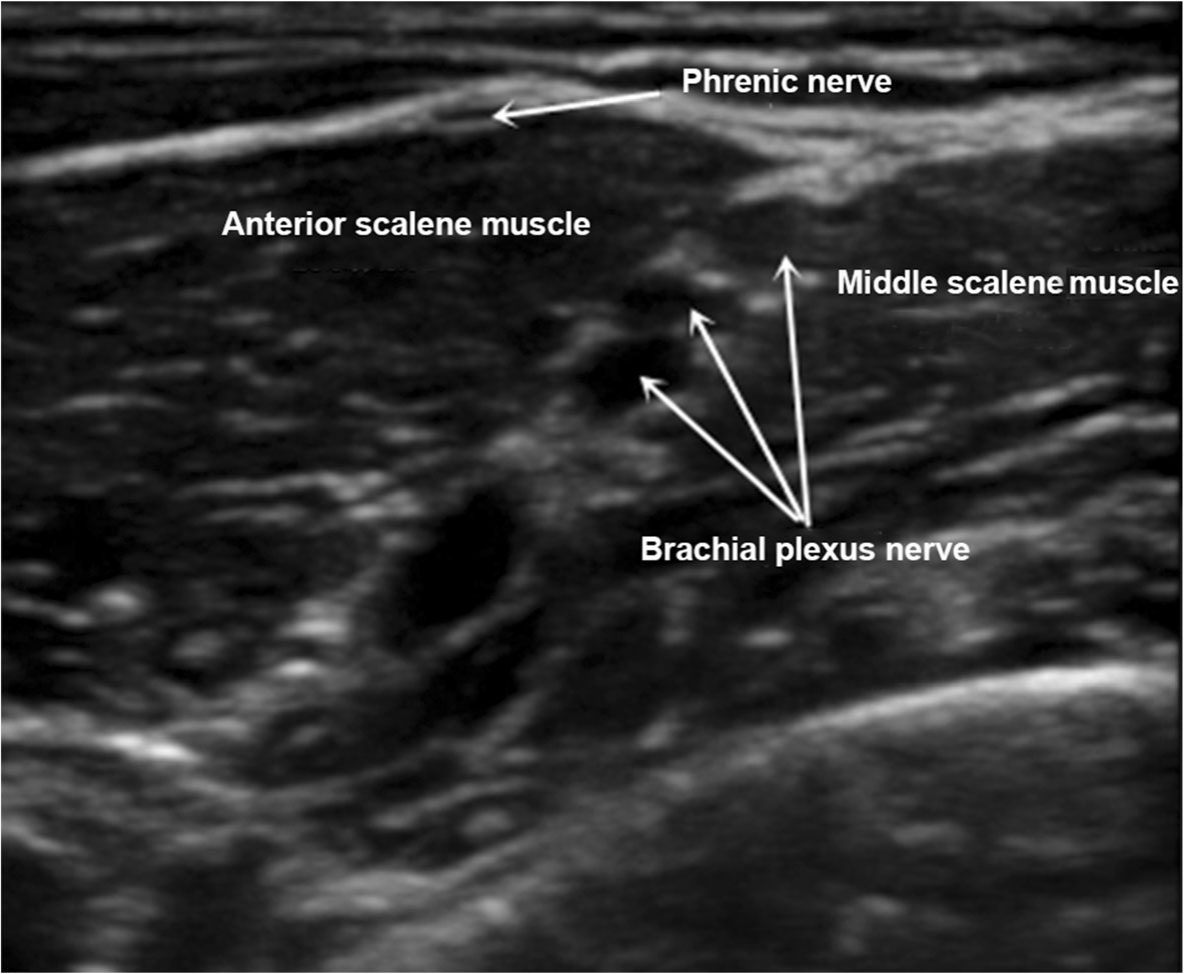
The phrenic nerve ultrasonography of the right neck
